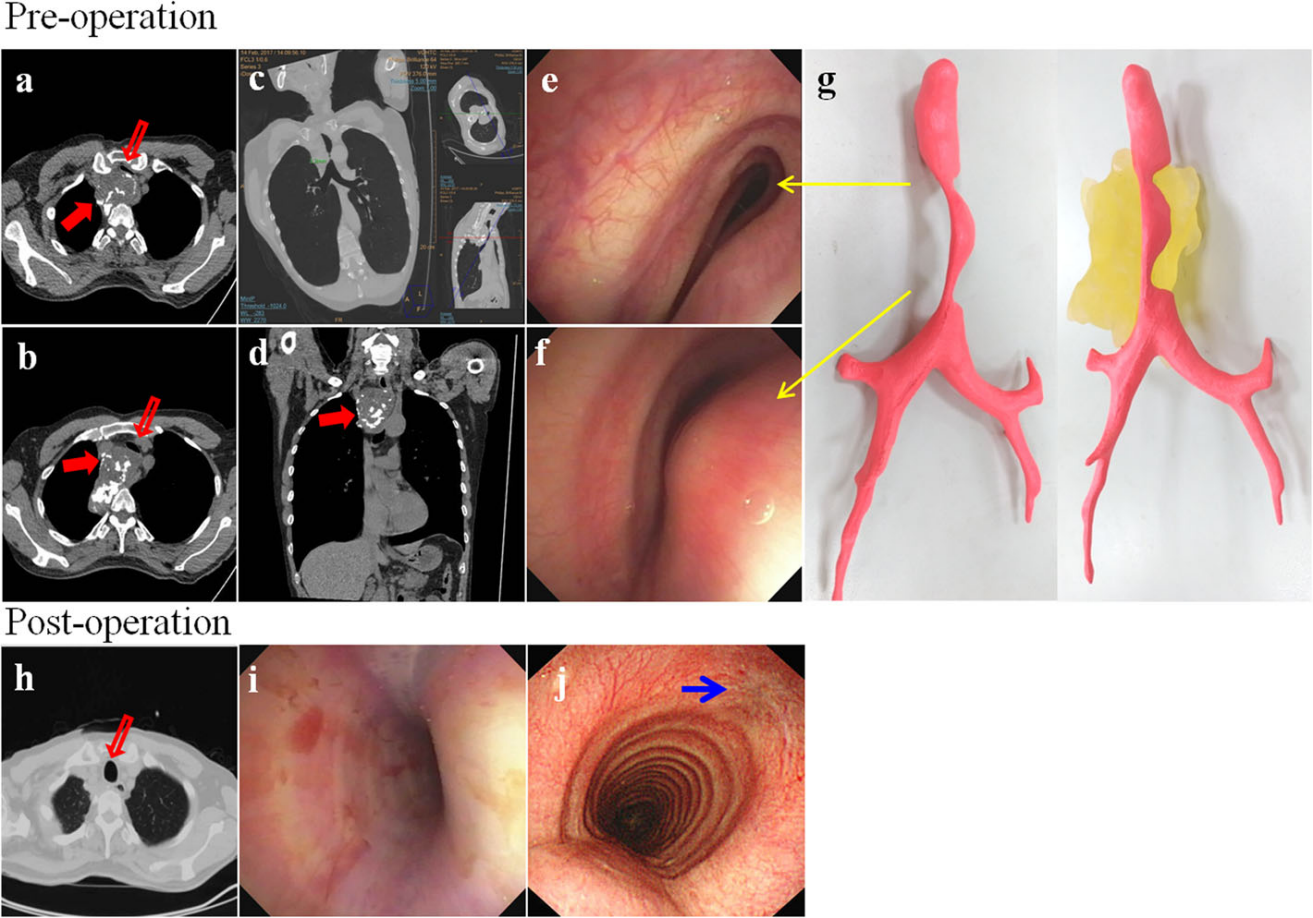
A noncontrast computed tomographic (CT) image shows severe compression of the esophagus and trachea by an approximately 8.3 × 7.5 × 4-cm lobulated tumor with multiple coarse calcifications at right upper mediastinum (a–d). Endoscopy shows a discrete compressed region of the trachea (e, f). The 3-D model of the trachea derived from CT images (g). The trachea moderately recovered after the insertion of a T-tube stent (i). CT scan and endoscopy of the trachea returned to normal after surgery (h, j). *Empty red arrows* mark the trachea; *solid red arrows* mark the chondrosarcoma; *yellow arrows* mark different sites of the trachea stenosis; *blue arrow* marks the site of tracheostomy
